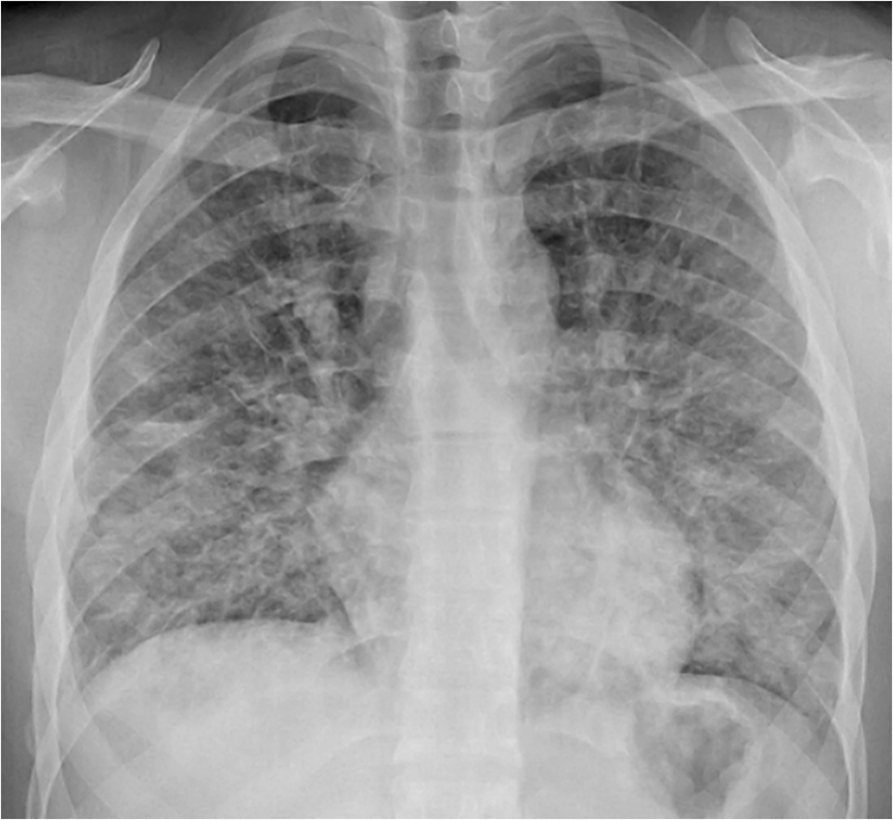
Chest X-ray at time of first admission shows diffuse diffuse reticulonodular pattern.
